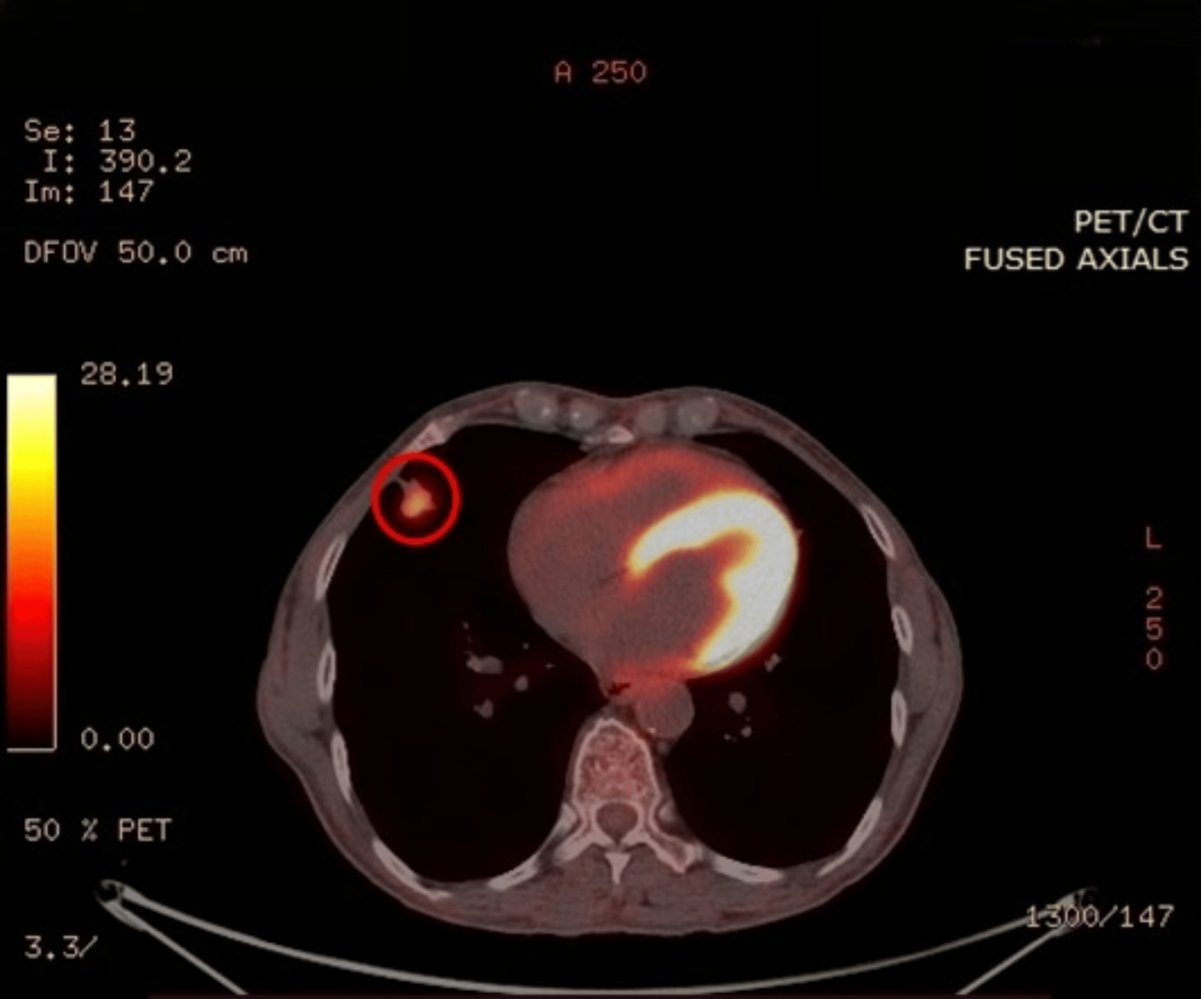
Metabolically Active Pulmonary Nodule on 18F-FDG PET-CT Scan 18F-FDG PET-CT image demonstrates the metabolically active 18 mm pulmonary nodule with a SUVmax of approximately 6, located in the lateral segment of the right middle lobe (red circle) 18F-FDG, 18F-fluorodeoxyglucose; PET-CT, positron emission tomography-computed tomography; SUVmax, maximum standardized uptake value
